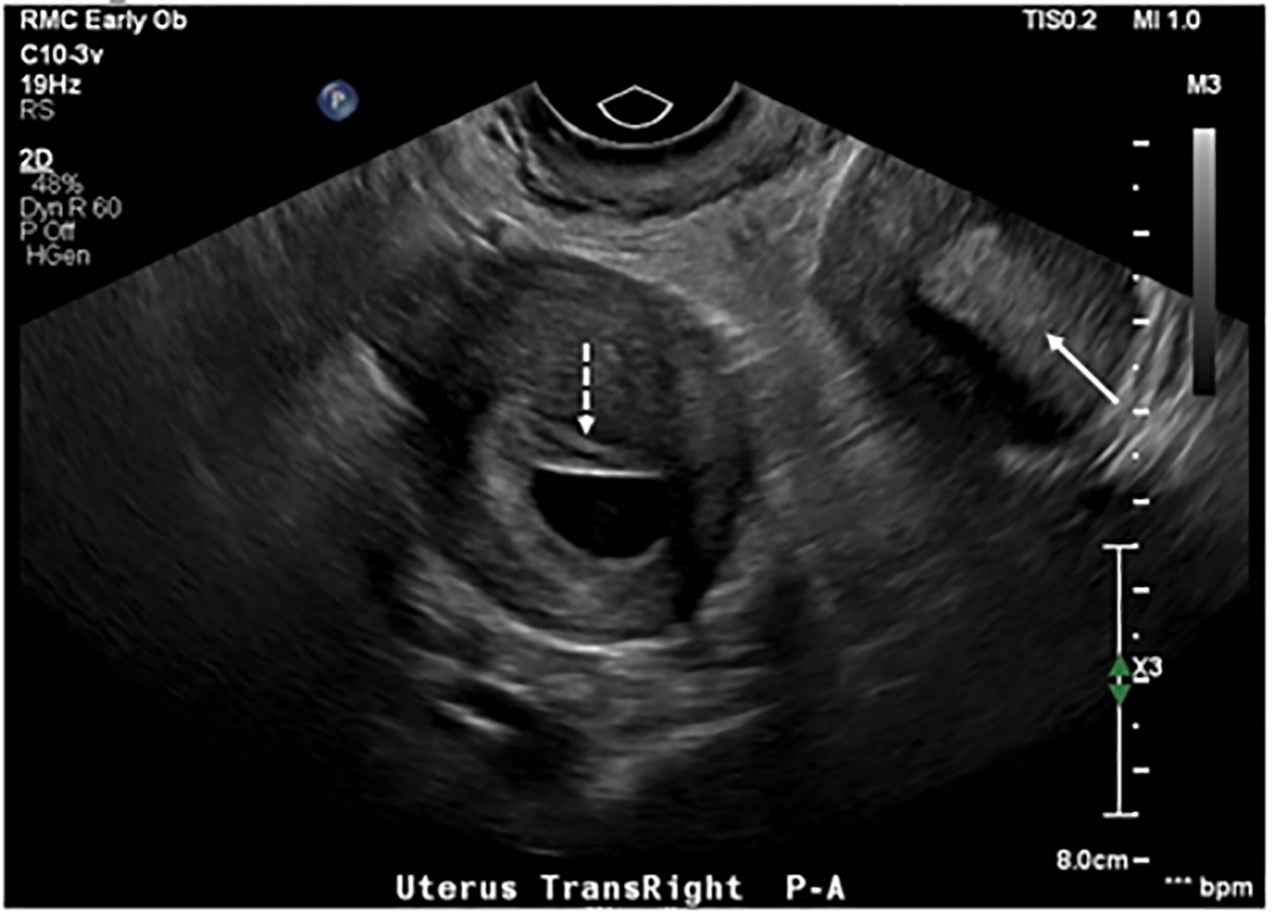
Transvaginal ultrasound. Transverse views of the uterus demonstrate uterus didelphys with both the right uterus (interrupted arrow) with gestation sac present and adjacent left uterus (solid arrow) with absence of gestation sac.
